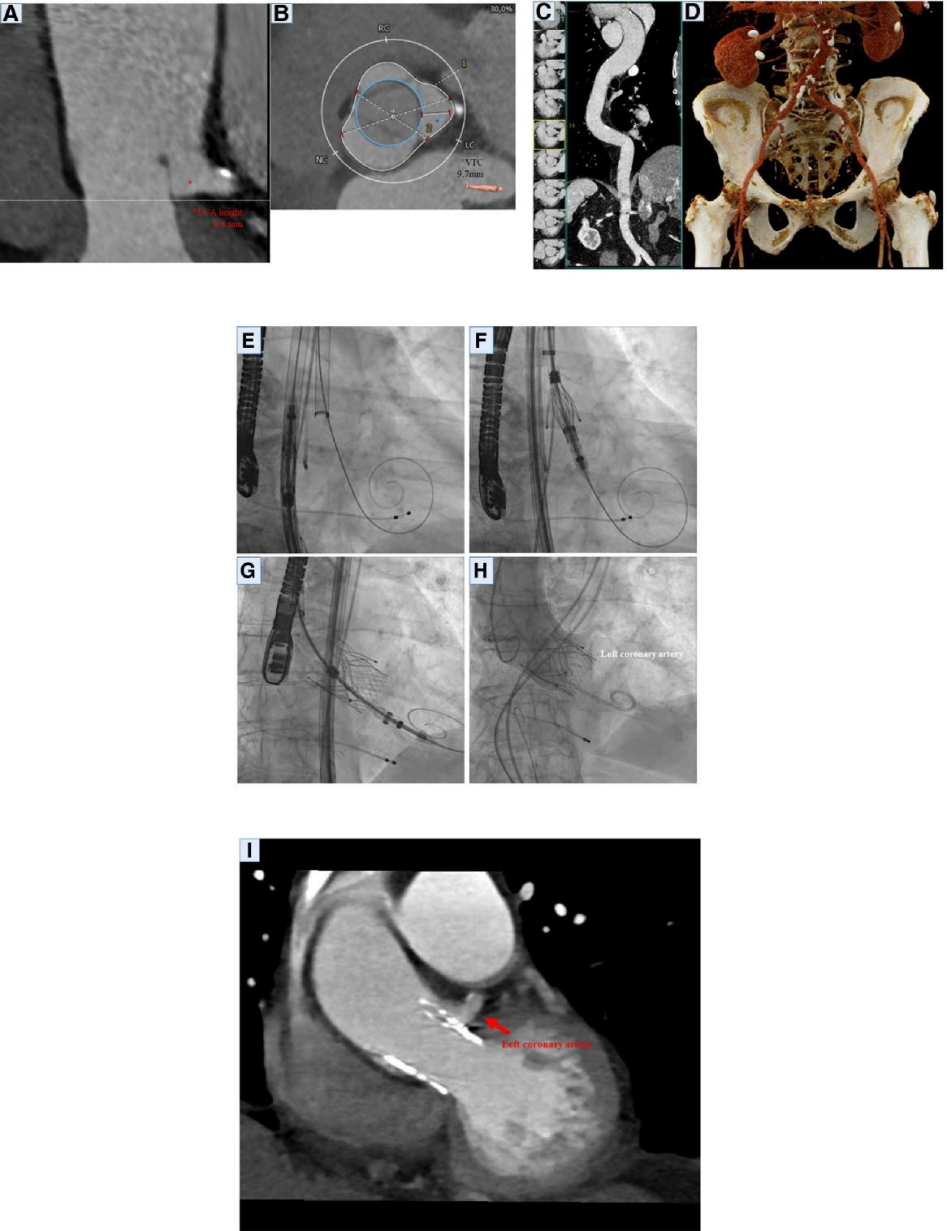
Computed tomography (CT) showing the device landing zone and the left coronary artery ostium height (*A*). Computed tomography depicting the virtual valve-to-coronary distance (*B*). Computed tomography angiography from the aortic valve to the descending aorta (*C*) and three-dimensional reconstruction of the femoral arteries (*D*). Angiography showing the valve delivery system in the ascending aorta (*E*), Trilogy valve prosthesis implantation under rapid pacing (*F* and *G*), and final angiography confirming left coronary artery perfusion (*H*). Computed tomography angiography (*I*) showing perfusion of the left coronary artery after transcatheter aortic valve implantation. LCA, left coronary artery; VTC, virtual valve-to-left coronary distance.
